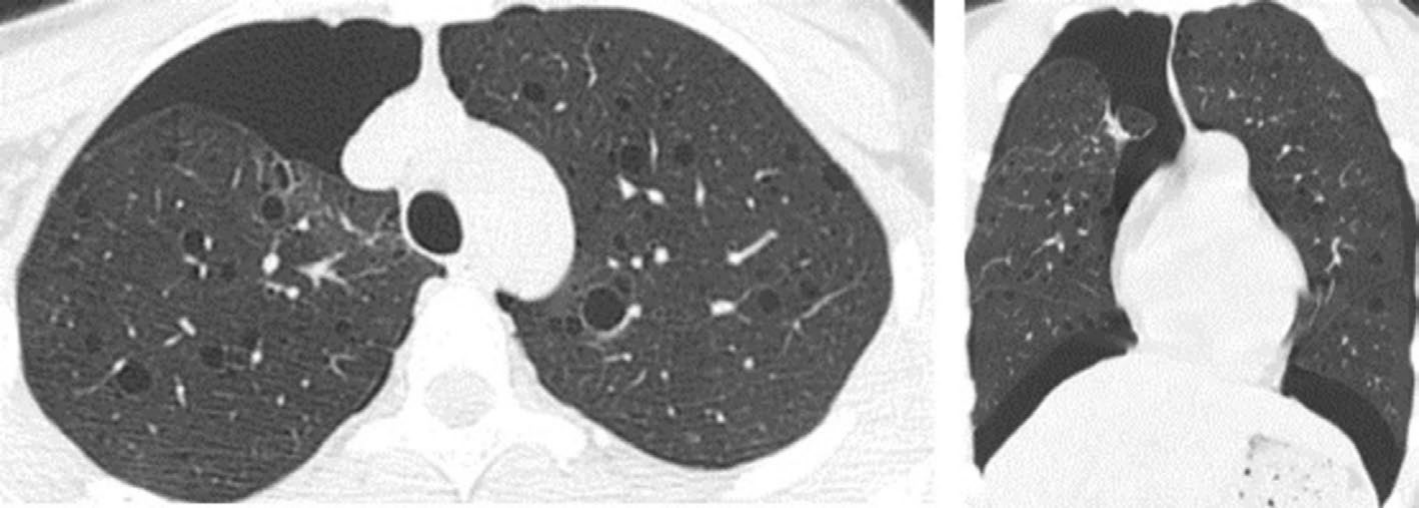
Chest CT scan obtained on April 25 demonstrates multiple cystic lesions in both lungs, bilateral fibrotic foci, and small pneumothoraces on both sides, with approximately 30% compression of the right lung and 20% compression of the left lung.
